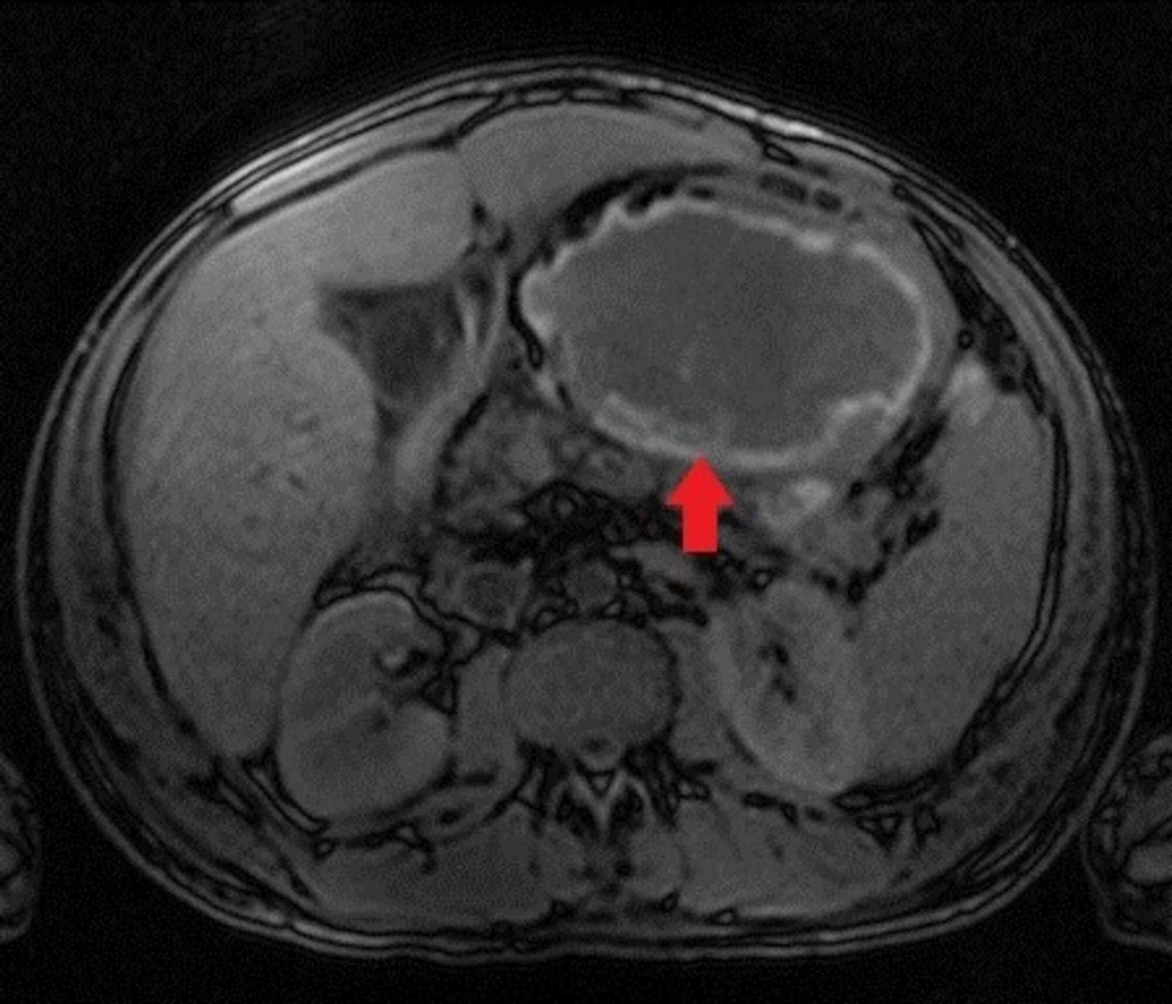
Axial MRI showing advancing encapsulated subacute intraperitoneal hematoma MRI: magnetic resonance imaging.
